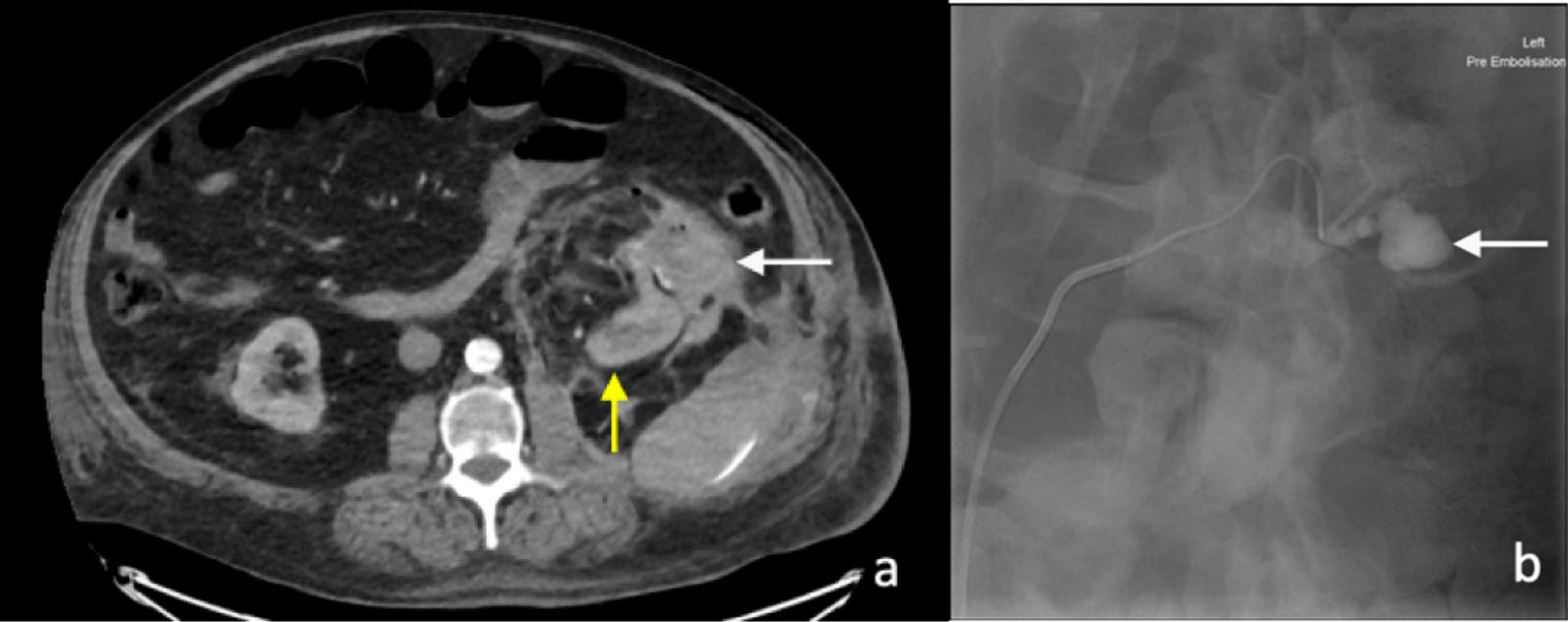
a Arterial phase CT image of perinephric haematoma (white arrow) at the site of partial nephrectomy. The residual left kidney is indicated by the yellow arrow. b Active extravasation of contrast (white arrow) was confirmed on DSA
